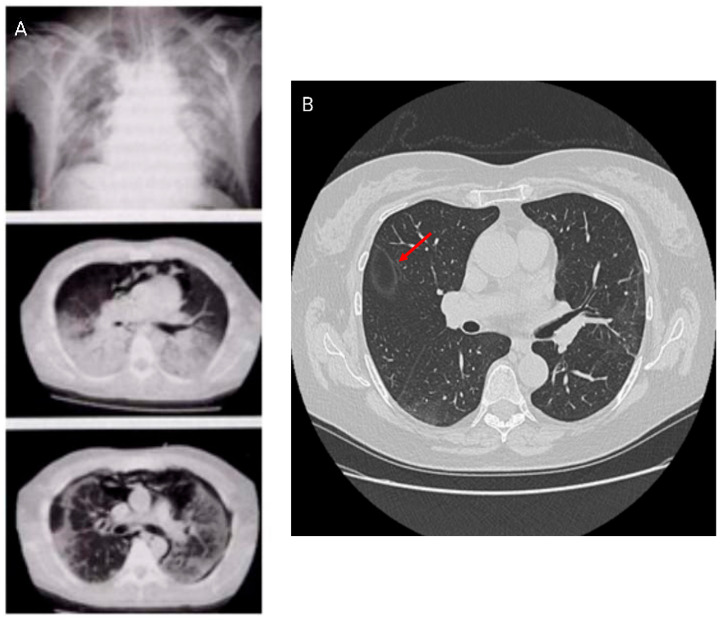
Radiological imaging in COVID-19 infection. HRCT patterns in Acute Respiratory Distress Syndrome (ARDS) (A) and in subacute phase with atoll or reversed halo sign ((B) arrow).
